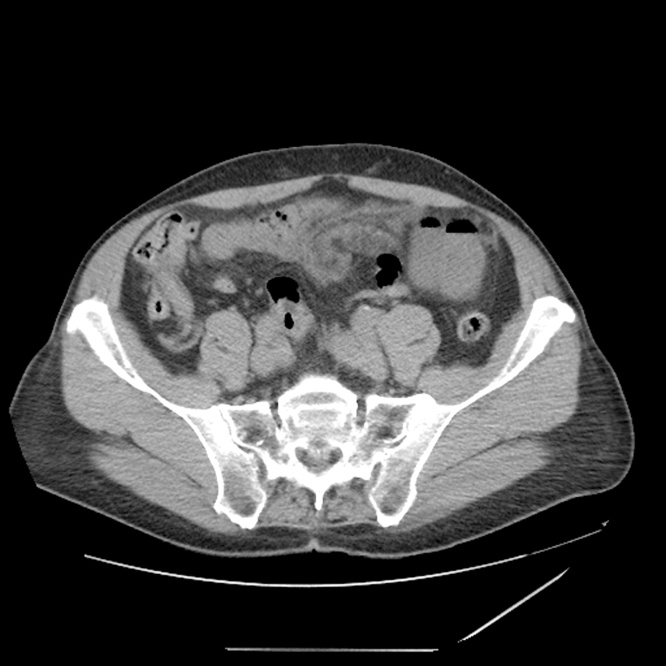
CT of the abdomen in a 78-year-old man with increasing abdominal pain over approximately 6 h. The CT scan shows whirling of the mid-abdominal mesentery with edematous changes of the fat and congestive prominence of mesenteric vessels amongst small bowel loops at this level. Early small bowel obstruction was seen with transition point in the left lower abdomen.
